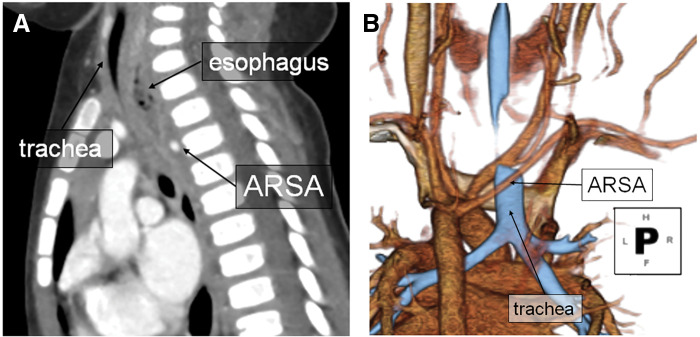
Images of CT scan on the 2nd hospital admission. The image reveals esophageal stenosis due to ARSA compression, along with esophageal dilatation proximal to the compression site. Additionally, the trachea appears narrowed due to compression from the dilated esophagus. (A) Sagittal view (B) 3D reconstruction image. ARSA, aberrant right subclavian artery
